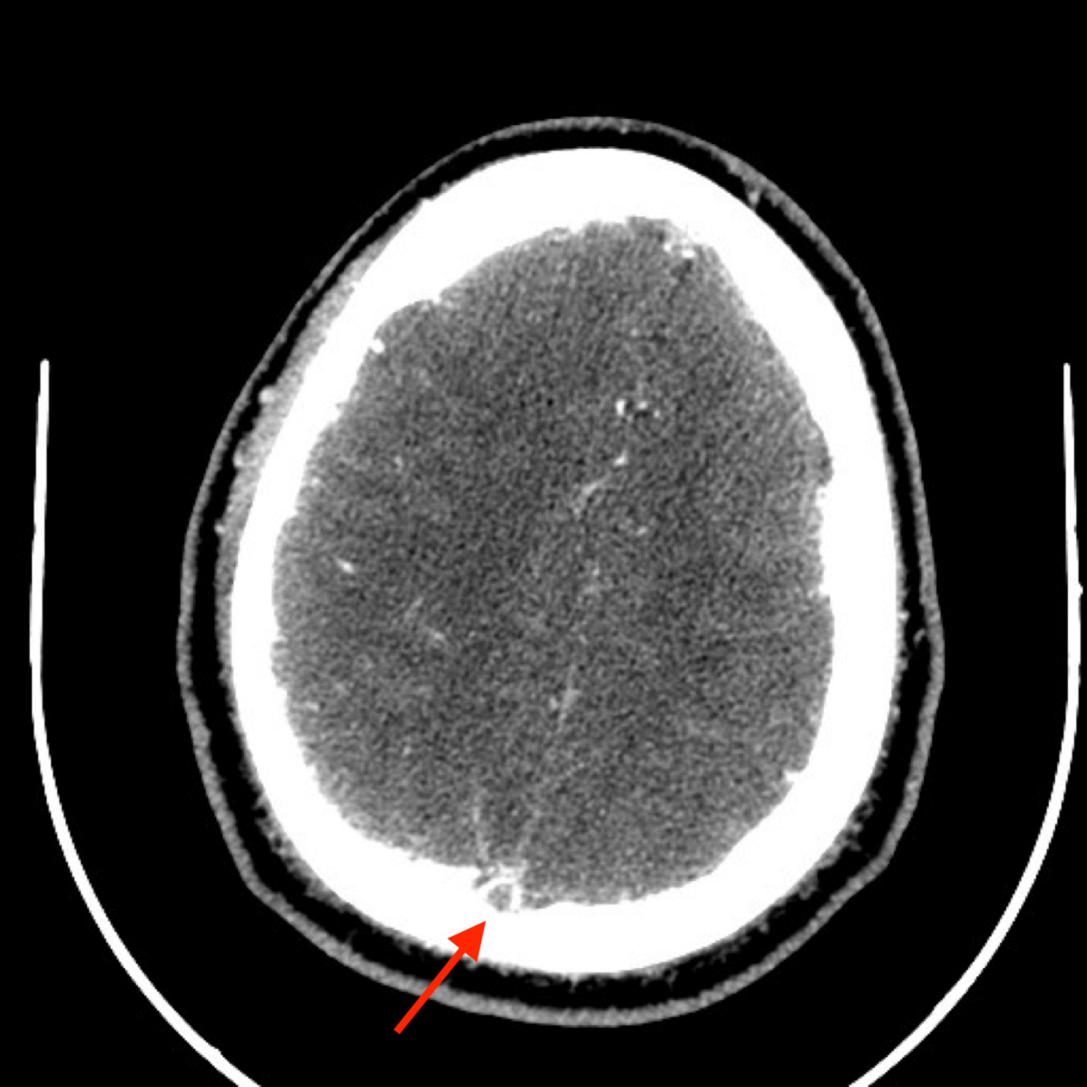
Axial computed tomography venography (CTV) of the thrombus demonstrating delta sign
